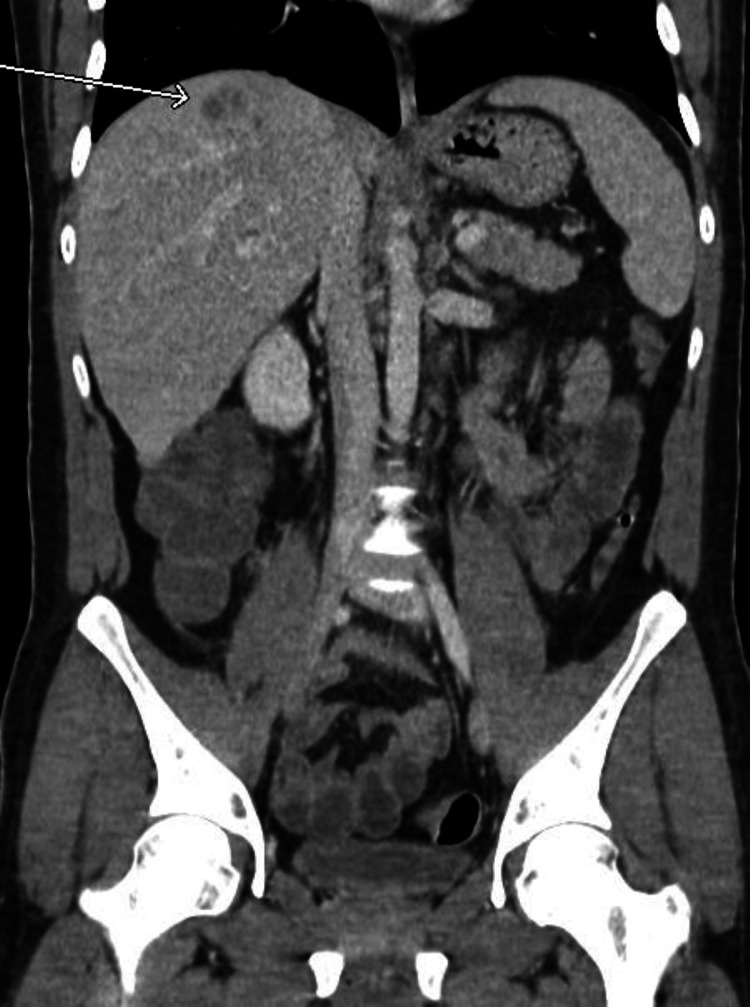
CT abdomen (coronal view) showing possible liver abscess
